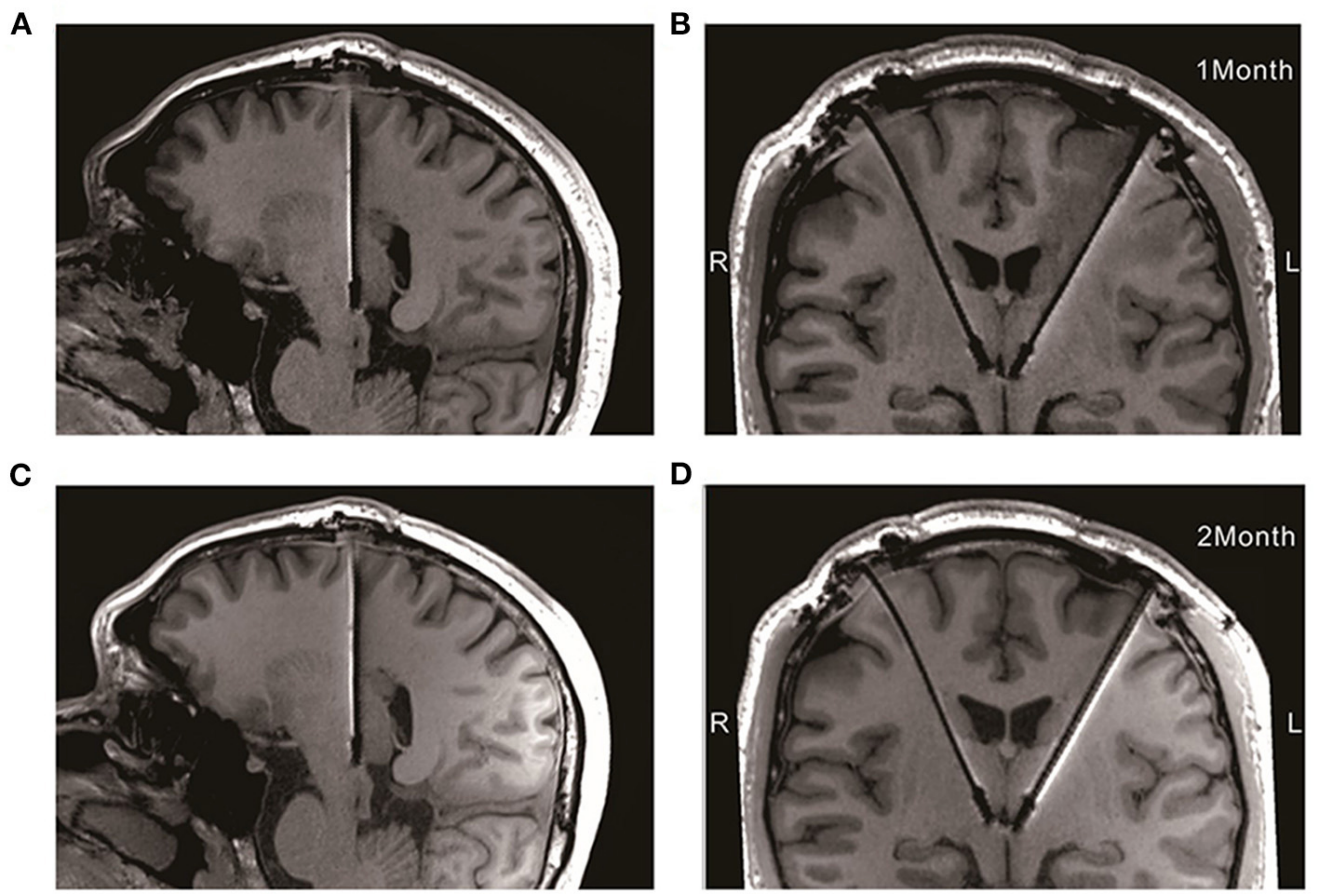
Electrodes placement at 1 and 2-month after implantation with 3T MRI. (A,B) The electrodes in the median sagittal and coronal section at 1-month after implantation, respectively; (C,D) The electrodes in the median sagittal and coronal section at 2-month after implantation, respectively.
